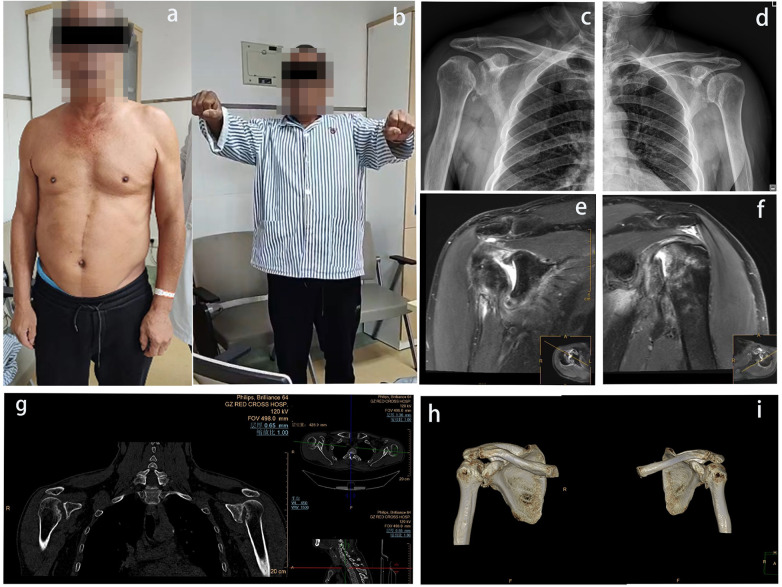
(a,b) A preoperative examination showing a limited shoulder range of motion; (c,d) preoperative anteroposterior (AP) radiographs; (e,f) an MRI demonstrating partial-thickness supraspinatus and subscapularis tendon tears with long head of the biceps tendon–superior labrum (SLAP complex) abnormalities; and (g–i) CT images showing a locked posterior dislocation of both shoulders with humeral head defects.
